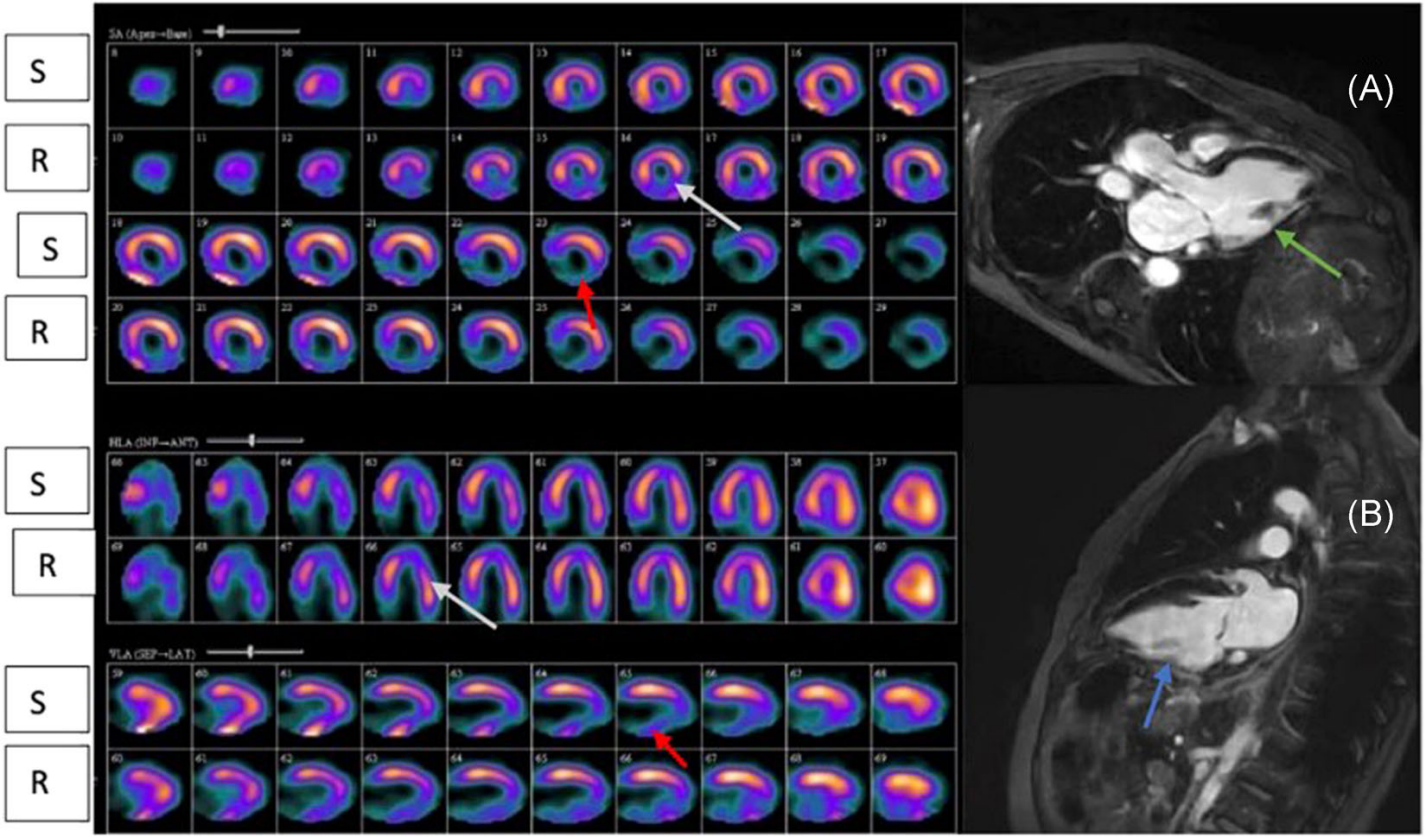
(A) PVM on SPECT showed subendocardial infarct on CMR. (B) NVM on SPECT confirmed as a transmural scar on CMR. (A) Inferolateral wall on SPECT that is partially reversible on rest imaging (gray arrow), suggestive of PVM; inferolateral wall on LGE of CMR reveal subendocardial infarct of <50% transmural extent (green arrow). (B) Inferior wall perfusion fixed defect on stress and rest SPECT imaging (red arrow), indicative of NVM and confirmed by CMR as transmural scar (blue arrow). CMR, cardiac magentic resonance; LGE, late gadolinium enhancement; NVM, nonviable myocardium; PVM, partially viable myocardium; R, rest attenuation corrected; S, stress attenuation corrected; SPECT, Single Photon Emission Computed Tomography; VM, viable myocardium
